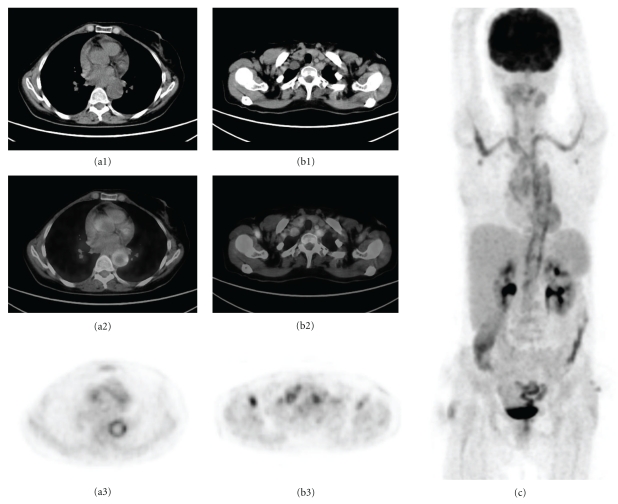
A 54-year-old woman with FUO underwent PET-CT for the diagnosis of underlying disease. Transaxial slices of CT (a1, b1), fusion (a2, b2), and PET (a3, b3) and anterior MIP image (c) showed accumulation of FDG in the wall of thoracic aorta and the supra-aortal branches. Gigantocellular arteritis was confirmed subsequently by temporal arterial biopsy.
